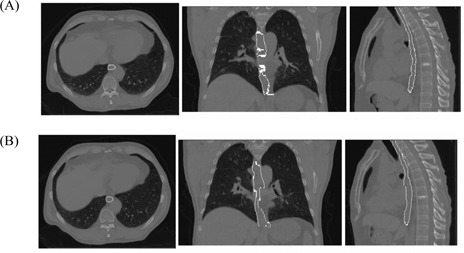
Esophagus contour from end‐expiration to end‐inspiration computed tomography (CT) images, mapped using optical flow. The two image sets were picked from the same location in the image coordinate system, not from exactly the same anatomic location. Panel A shows the original esophagus contour on end‐expiration CT. Panel B shows the mapped esophagus contour on end‐expiration CT.
